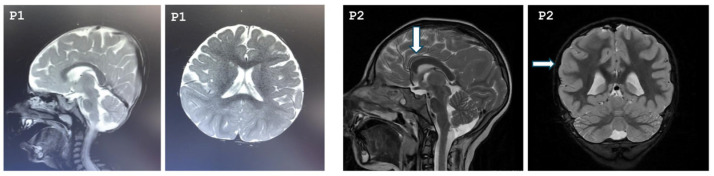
Cranial MRI images of Patient 1 and Patient 2. P1: T2-weighted sagittal and axial cranial MRI of Patient 1 demonstrating bilateral diffuse cerebral atrophy. P2: The arrows indicate a hypoplastic corpus callosum and pachygyria, respectively, in Patient 2.
